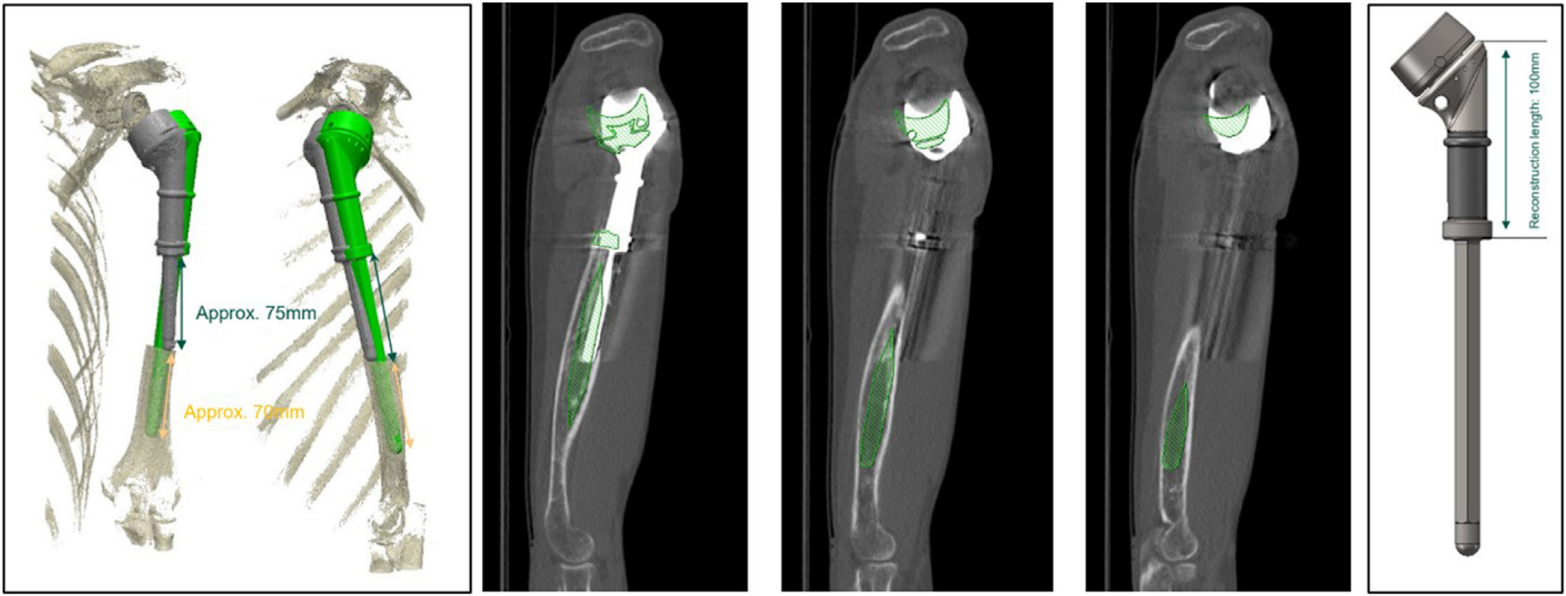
CT reconstruction and preoperative planning for the ordering of a longer custom-made prosthesis. *CT*, computed tomography.
